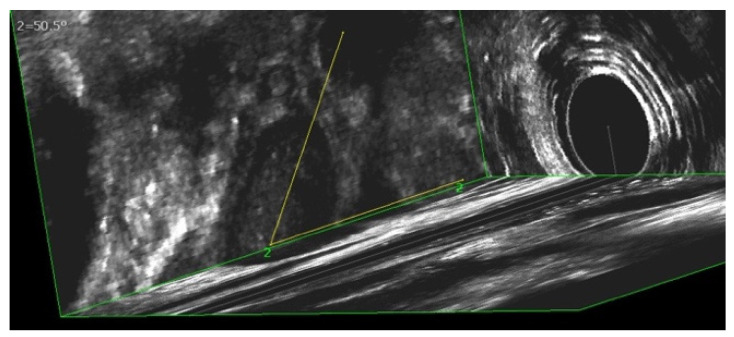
3D-endoanal ultrasound image: A migrated prosthesis in a 50.5° angle to the anal canal.
